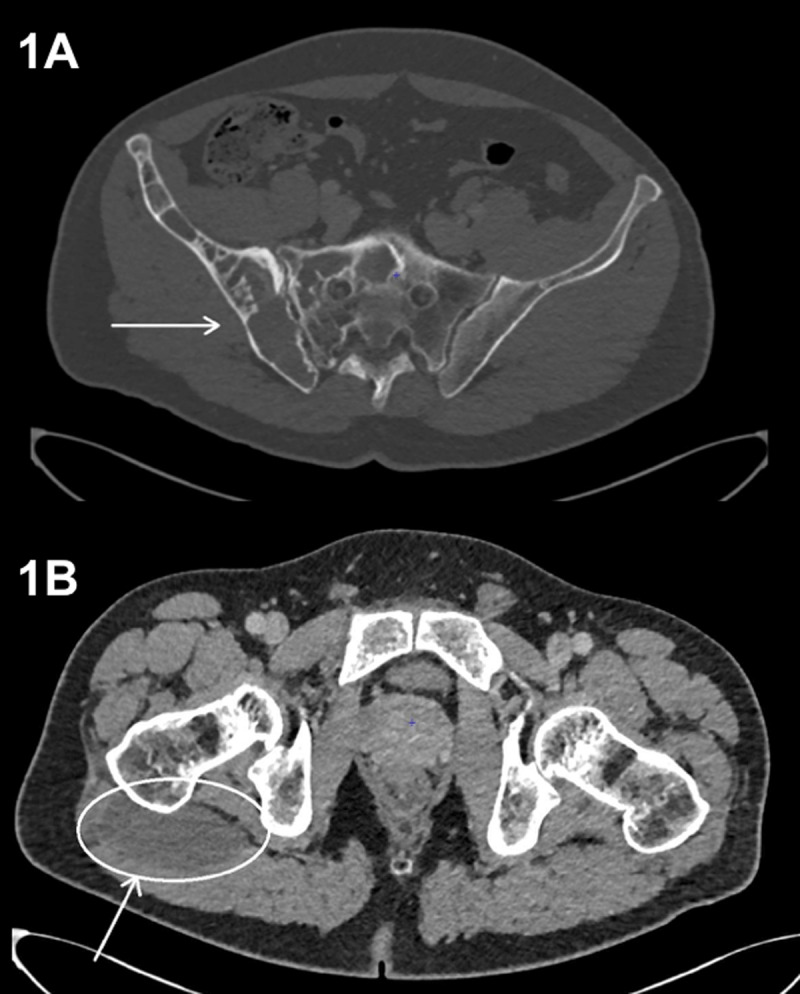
As the patient indicated intermittent right gluteal and lumbar pain over the last months without any functional impairment, pelvic magnetic resonance imaging (MRI) was performed for characterization.
